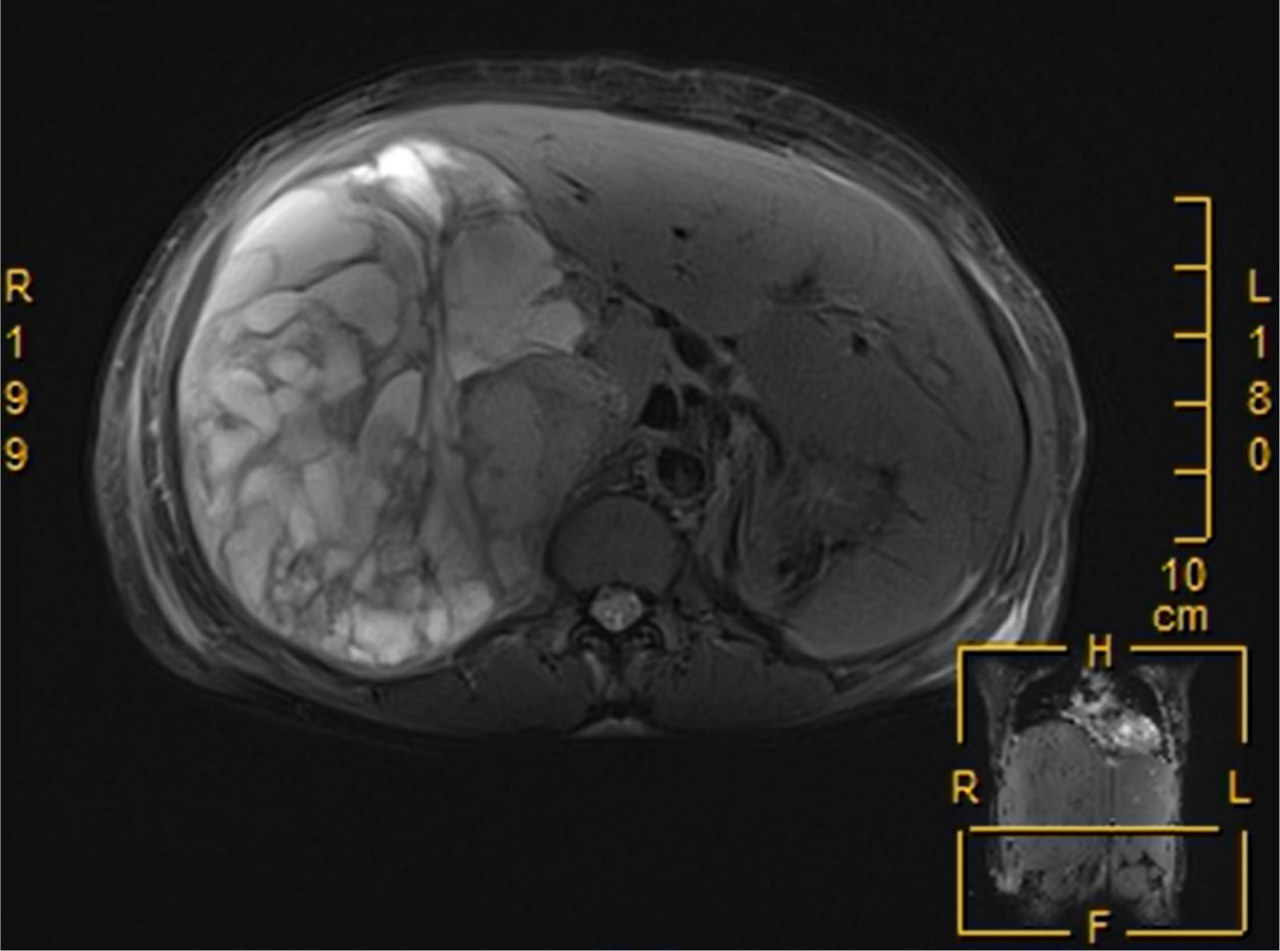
T2-weighted fat-suppressed magnetic resonance imaging predominantly revealed mixed high signal intensities.
